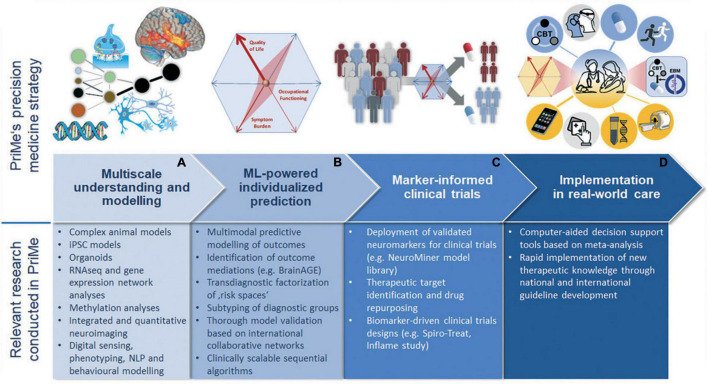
Concept figure representing the translational chain from understanding over development of predictive tools to their translation into clinical practice through stratified clinical trials (A–D). The upper part depicts the envisaged bench-to-bedside sequence, while the lower part lists relevant research activities at each translational step that are being pursued by PriMe. iPSC, induced pluripotent stem cells; ML, machine learning; MRI, magnetic resonance imaging; NLP, neurolinguistic programming; NTBS, non-invasive transcranial brain stimulation; and PriMe, Precision in Mental Health.
